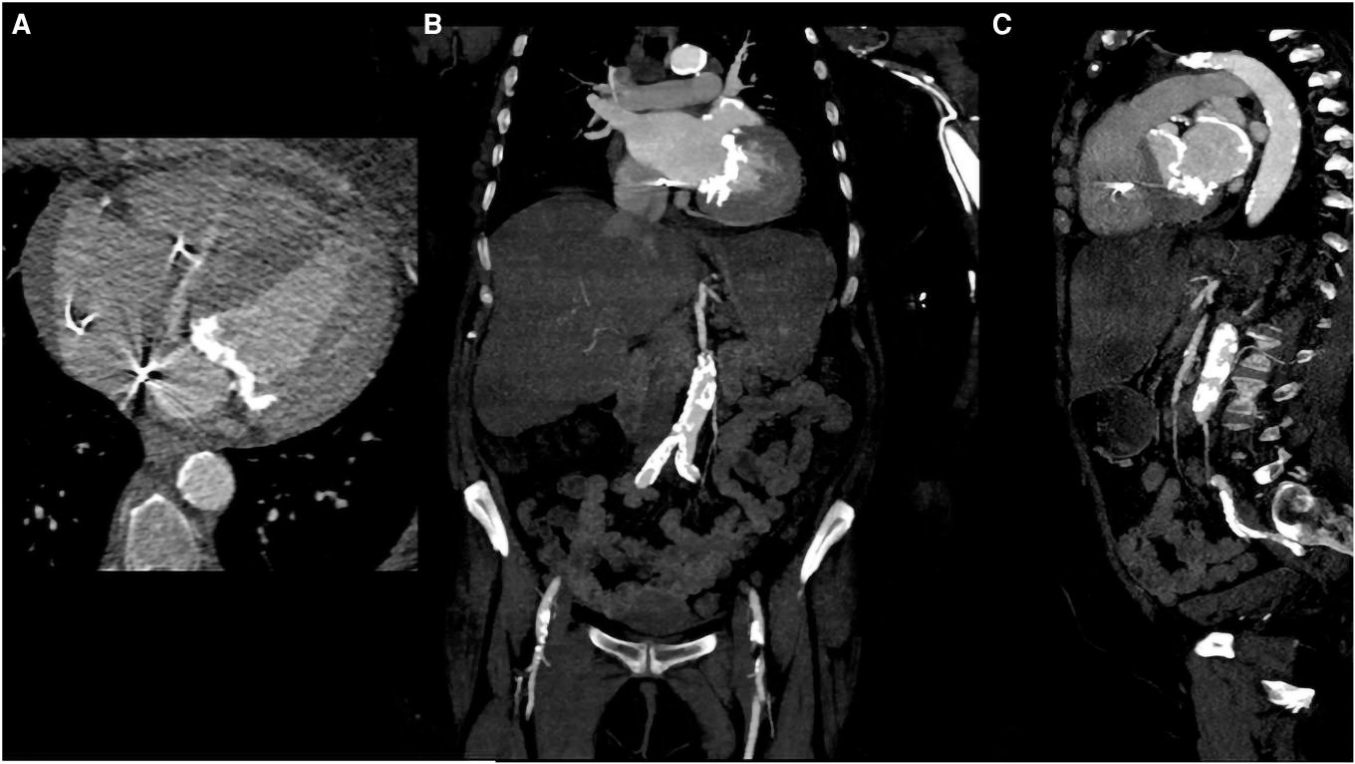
This cardiac CT image shows explicit calcifications within the heart. Main sites of calcifications are located at the site of the mitral annulus and involve a part of the nearby atrial wall. Panel *A*, axial view showing wide mitral calcification; panel *B*, coronal view; panel *C*, sagittal view, displaying extensive calcifications within left atrium, left ventricle, and mitral valve apparatus.
